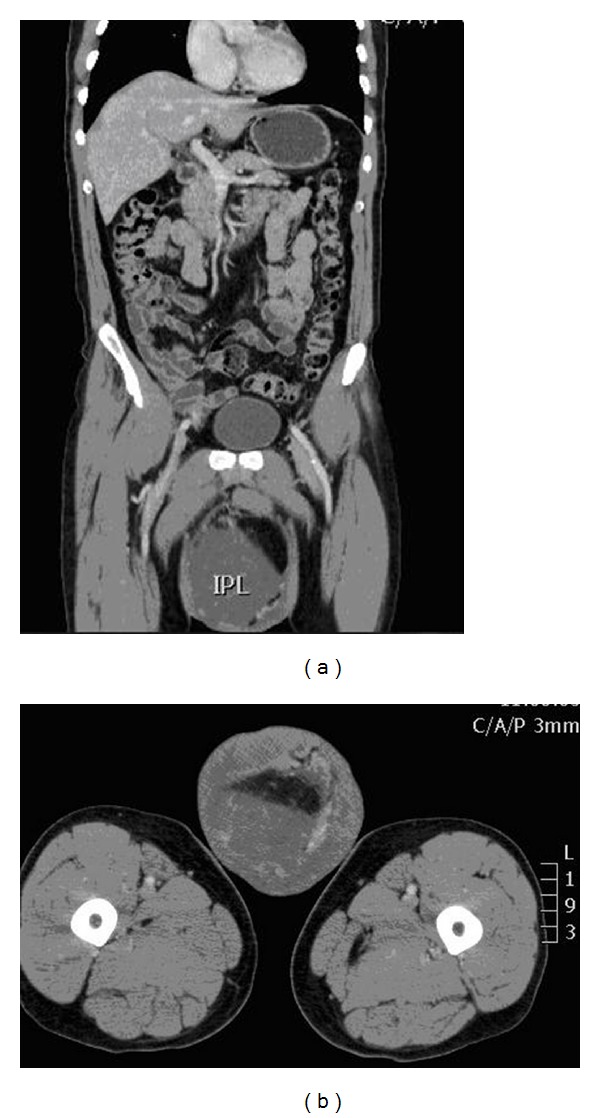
Coronal and axial views of CT abdomen and pelvis (a and b) showing the heterogenous mass with fat component.
